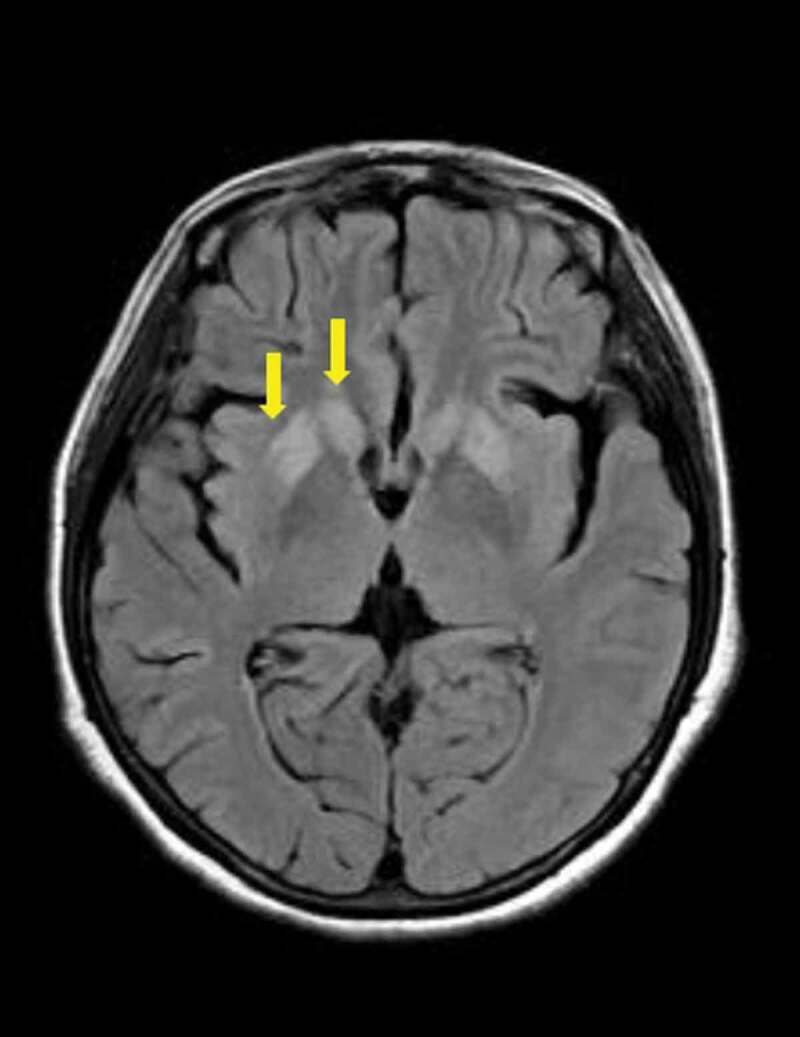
MRI with T2-FLAIR image of the patient.
